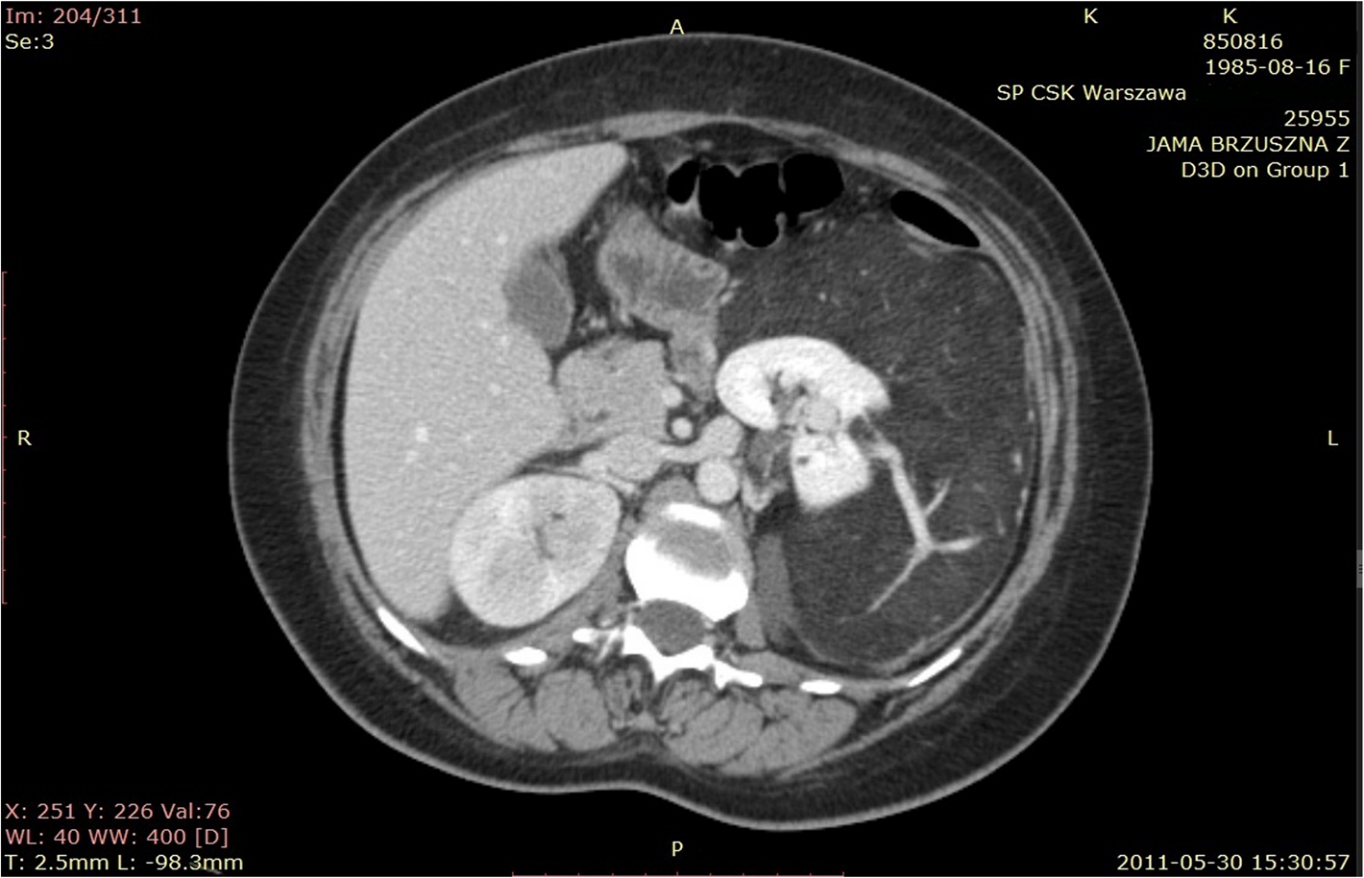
Computer tomography (CT) angiography - visible tumor and pathological vessel.
